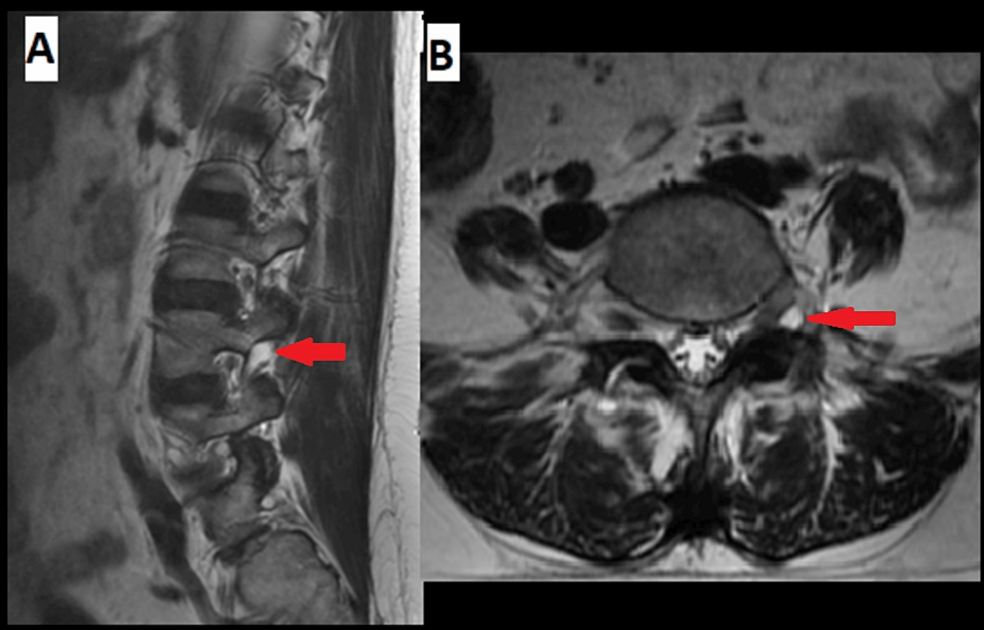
(A) Sagittal and (B) axial MRI showing the facet cyst (red arrow).
